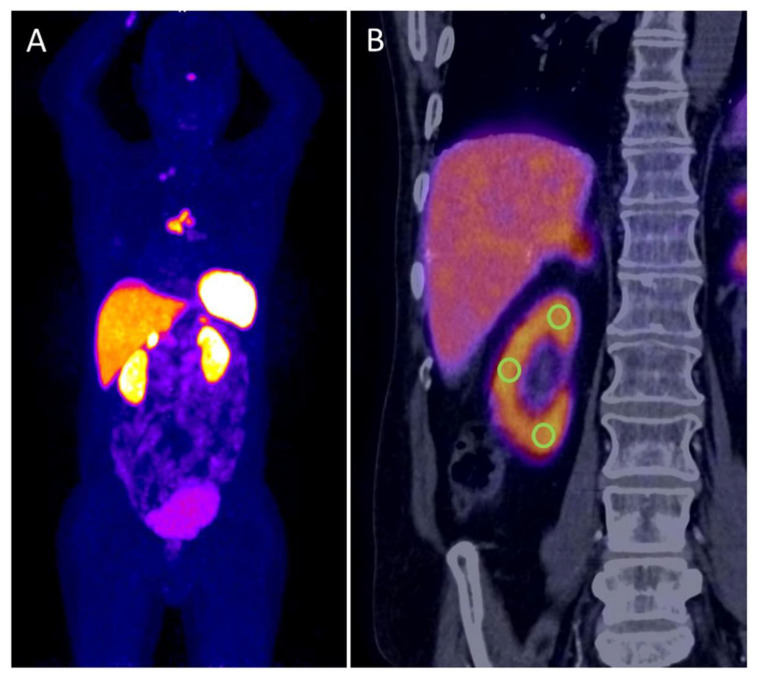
Maximum intensity projection of ^68^Ga[Ga]-DOTATOC PET with high tracer uptake in intrathoracic tumor manifestations, kidneys and typical SSTR-expressing organs (A). PET/CT coronal view of right kidney is showing the uptake in renal cortex and the localization of the three defined VOIs for SUV_mean_ measurements, which were averaged with measurements of the contralateral kidney (B).
